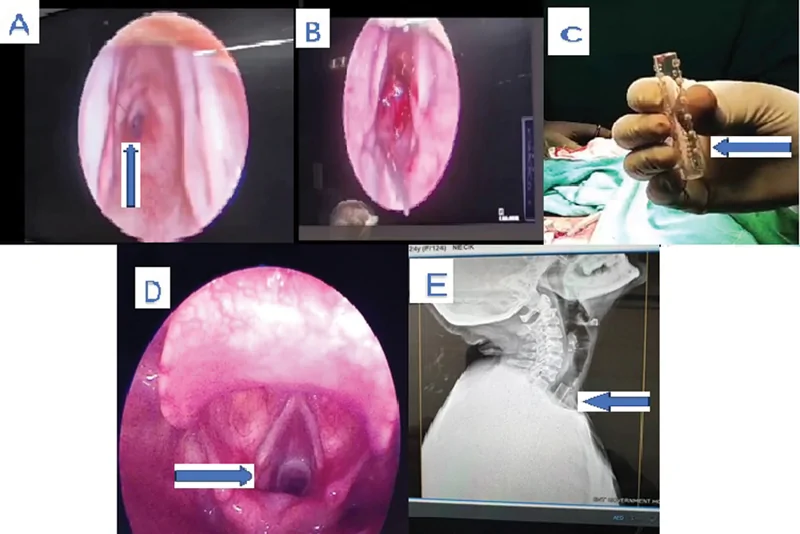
(A) Stenotic Segment; (B) After Bronchoscopic Dilatation; (C) Dumon stent with studs; (D) After placing Stent in Trachea; (E) Radiograph Neck Lateral View at 3-months follow-up showing stent in situ.
